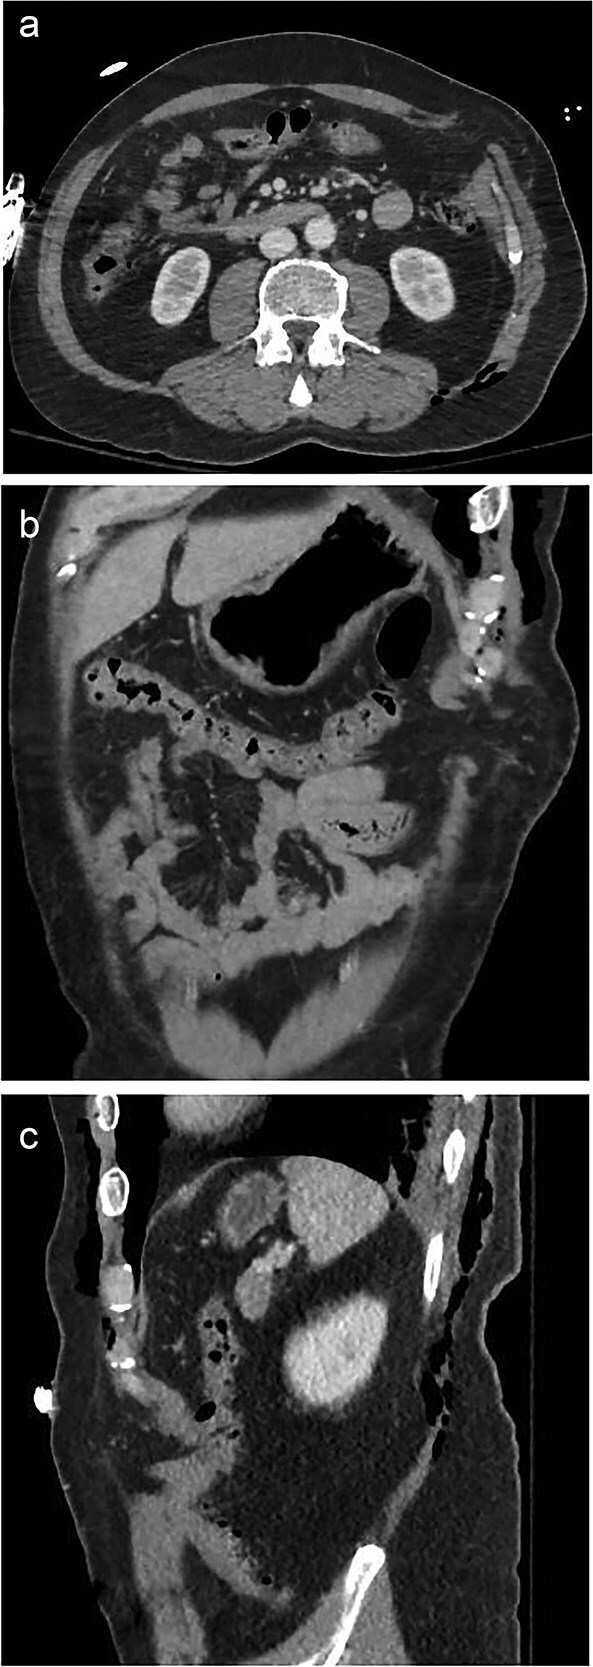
A 59-year-old male with a traumatic Spigelian hernia seen on CT. (a) Axial, (b) coronal, and (c) sagittal views of the hernia.
